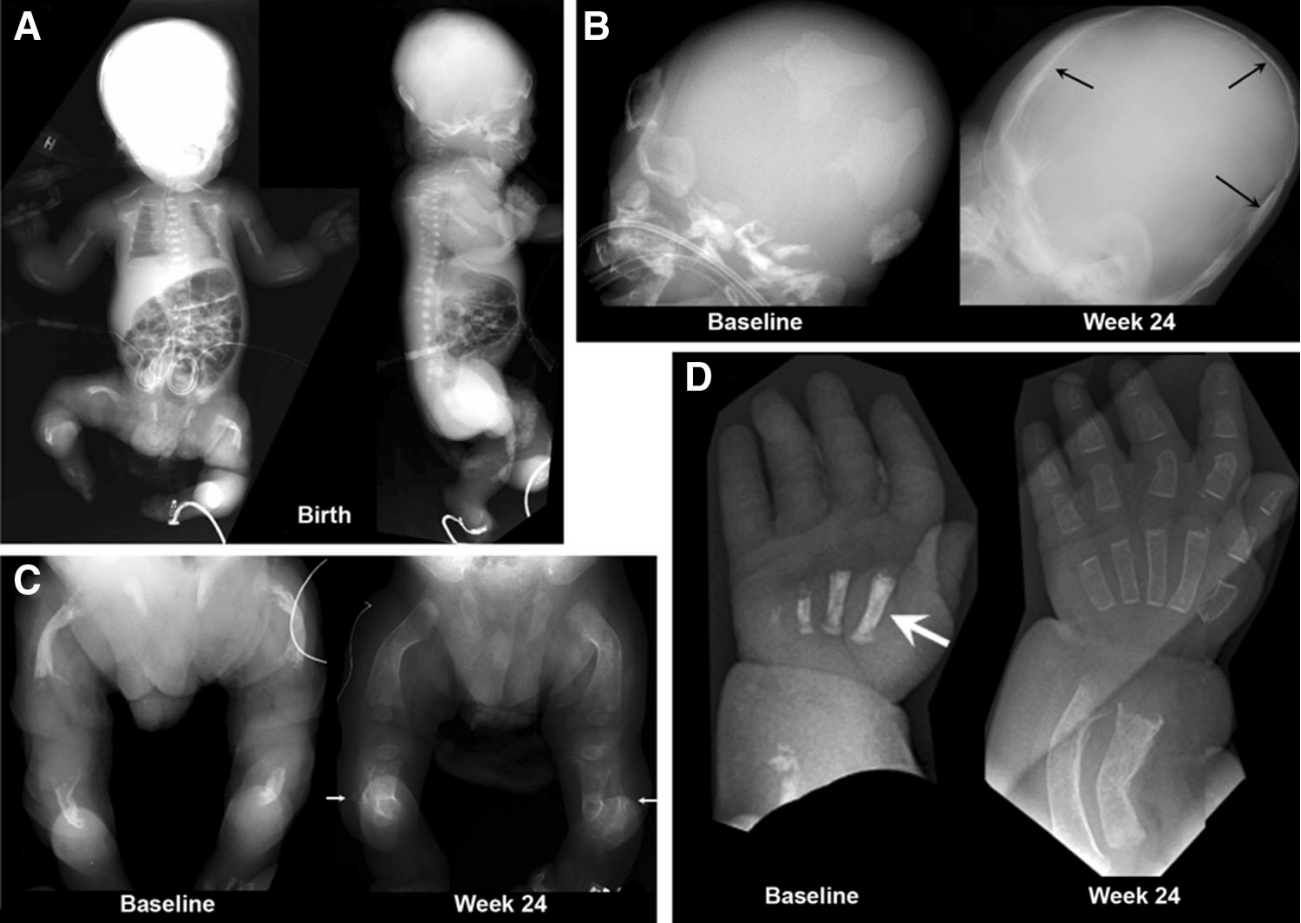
Efficacy of asfotase alfa in a 20-day-old (at therapy baseline) patient with life-threatening HPP. a This boy had shortened and bowed extremities and “fractures” detected by prenatal sonography at 17–18 weeks gestation. b Before asfotase alfa treatment, little or decreased mineral was present in the frontal, parietal, or occipital bones, skull base, facial bones, and sphenoid. c Before asfotase alfa, the femora were short, sclerotic, bowed, irregular, and lacked defined medullary cavities, cortices, and mineralized metaphyses and epiphyses. The fibulae were not calcified. After therapy, striking mineralization was evident. At week 24 of therapy, all areas showed striking remineralization. d The improvement in the left hand and wrist was remarkable. Images taken for the online supplementary data in [9] and reproduced with permission from The New England Journal of Medicine
